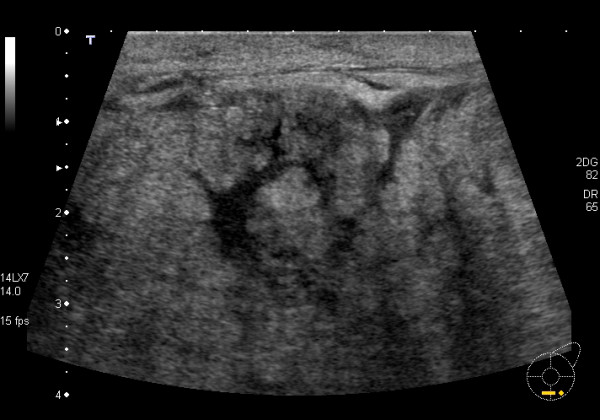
Mastitis of a lactating breast. The ultrasonic distinction of normal breast tissues is not evident. The parenchyma is markedly echogenic, ducts are non compressible and the skin lymphatics are visible. In addition the skin is thickened.
